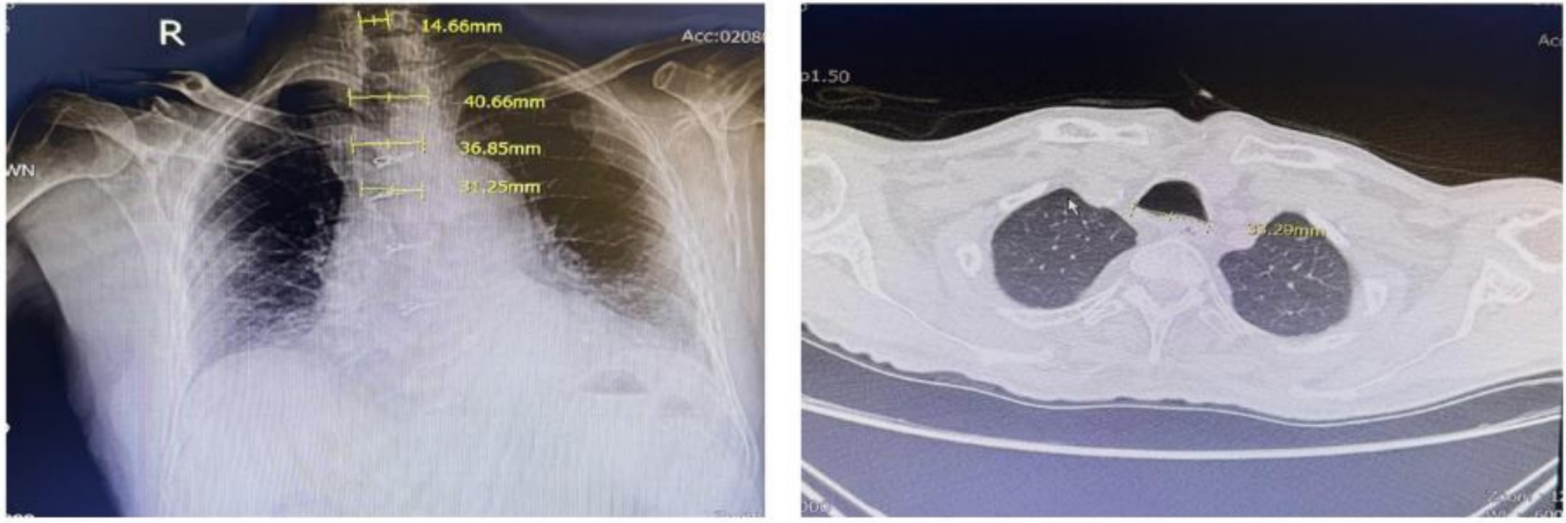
The preoperative chest x‐ray. The cross‐section chest CT image of the trachea, the trachea's maximum transverse diameter measured 33.29 mm.
